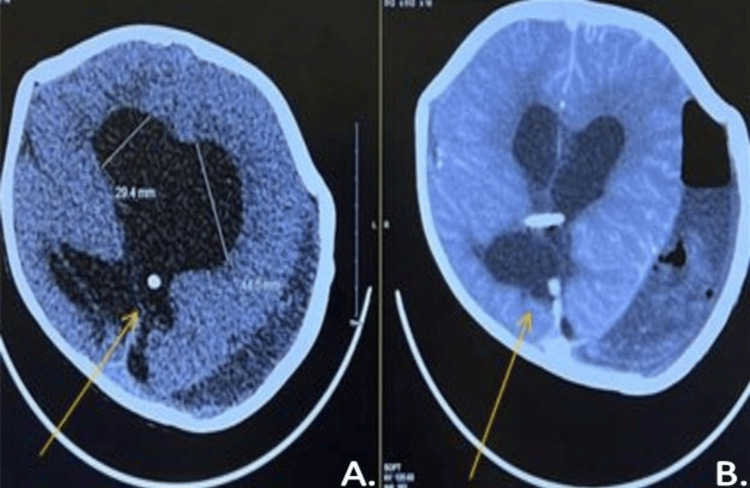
New and old brain CT. Comparison of the new brain CT (A) with the previous brain CT (B), which was done six months ago, shows a left frontal approach VP shunt with its tip seen at the occipital horn of the right lateral ventricle. There is an interval increase in the size of the supratentorial ventricular system.
